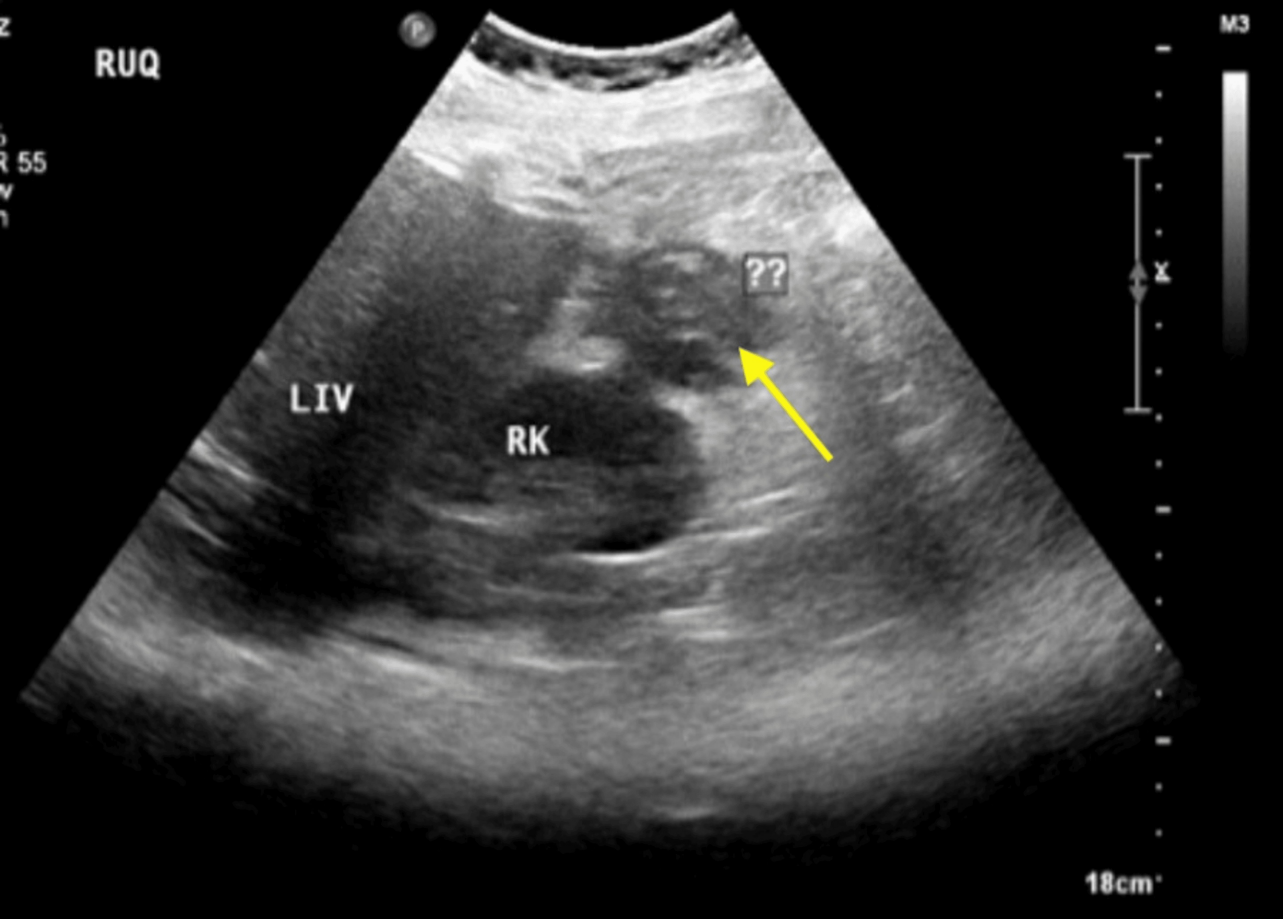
Ultrasonography of the abdomen. Ultrasonography of the abdomen demonstrating the ill-defined anechoic to hypoechoic structure adjacent to the right hepatic lobe with internal echogenicity.
